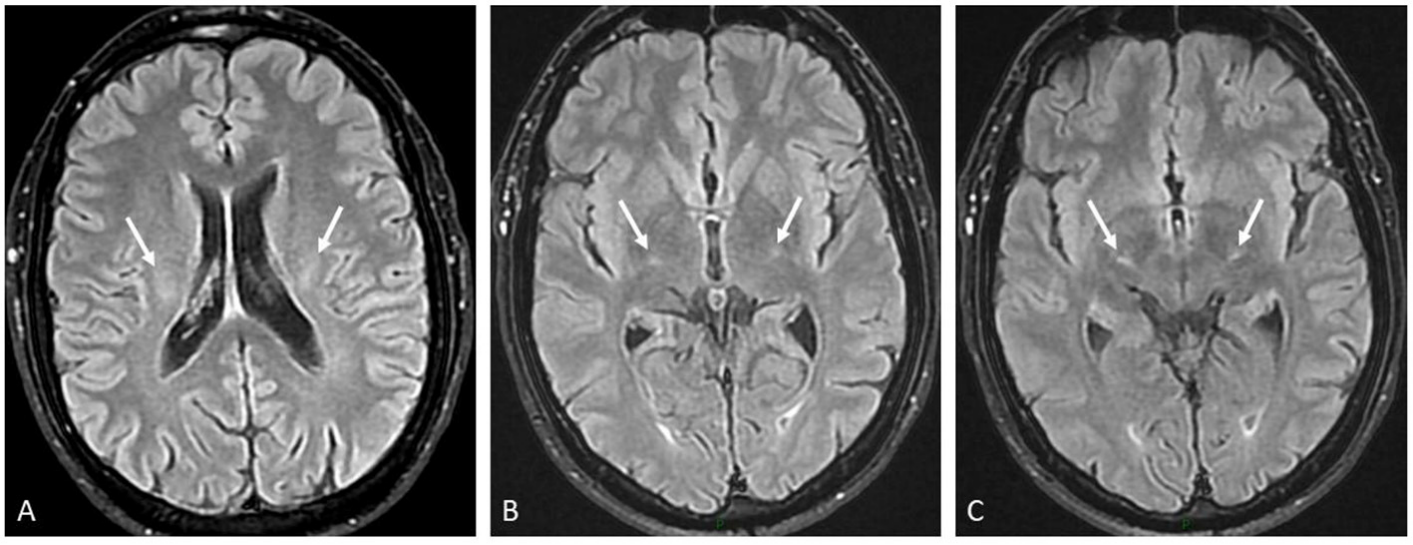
Brain MRI workup. Three axial transverse FLAIR views from the top to bottom showing moderate and symmetrical hypersignal intensity of the corticospinal tract (arrows) at the levels of the following: (A) the corona radiata, (B) the posterior arm of the internal capsule, and (C) the thalamo-mesencephalic junction.
